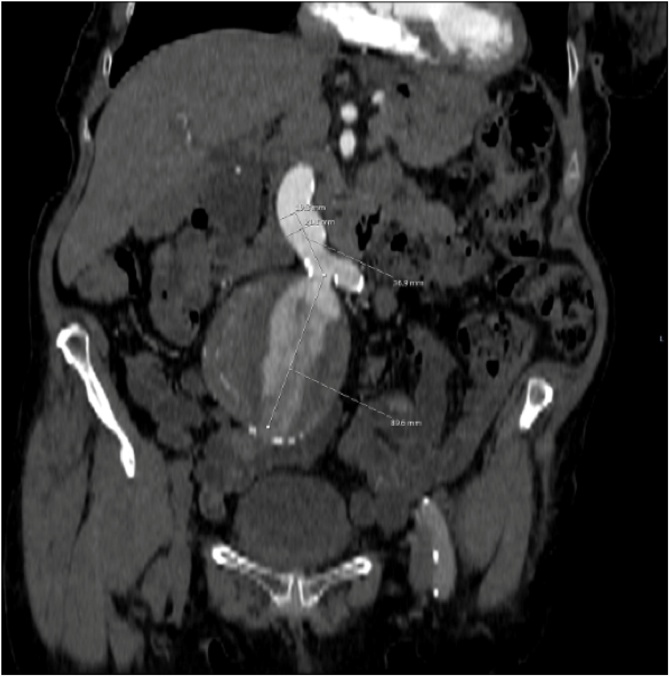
Coronal view, CT Abdomen Pelvis demonstrating normal Aorta and left Common Iliac Artery and Aneursymal Right Common Iliac Artery.
